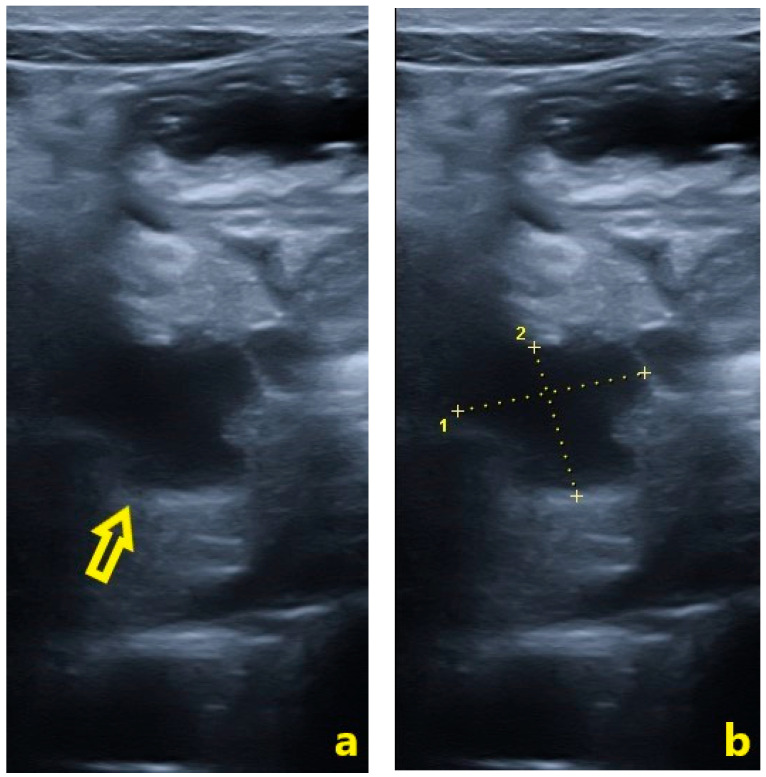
Abdominal ultrasound performed on day 10 post-injury. (a) The open yellow arrow indicates a well-defined, homogeneous peripancreatic fluid collection anterior to the pancreatic head, with progressively clearer margins compared to prior examinations, consistent with advancing organization. (b) Calipers indicate the collection’s dimensions: 20 × 16 mm (TS × AP).
